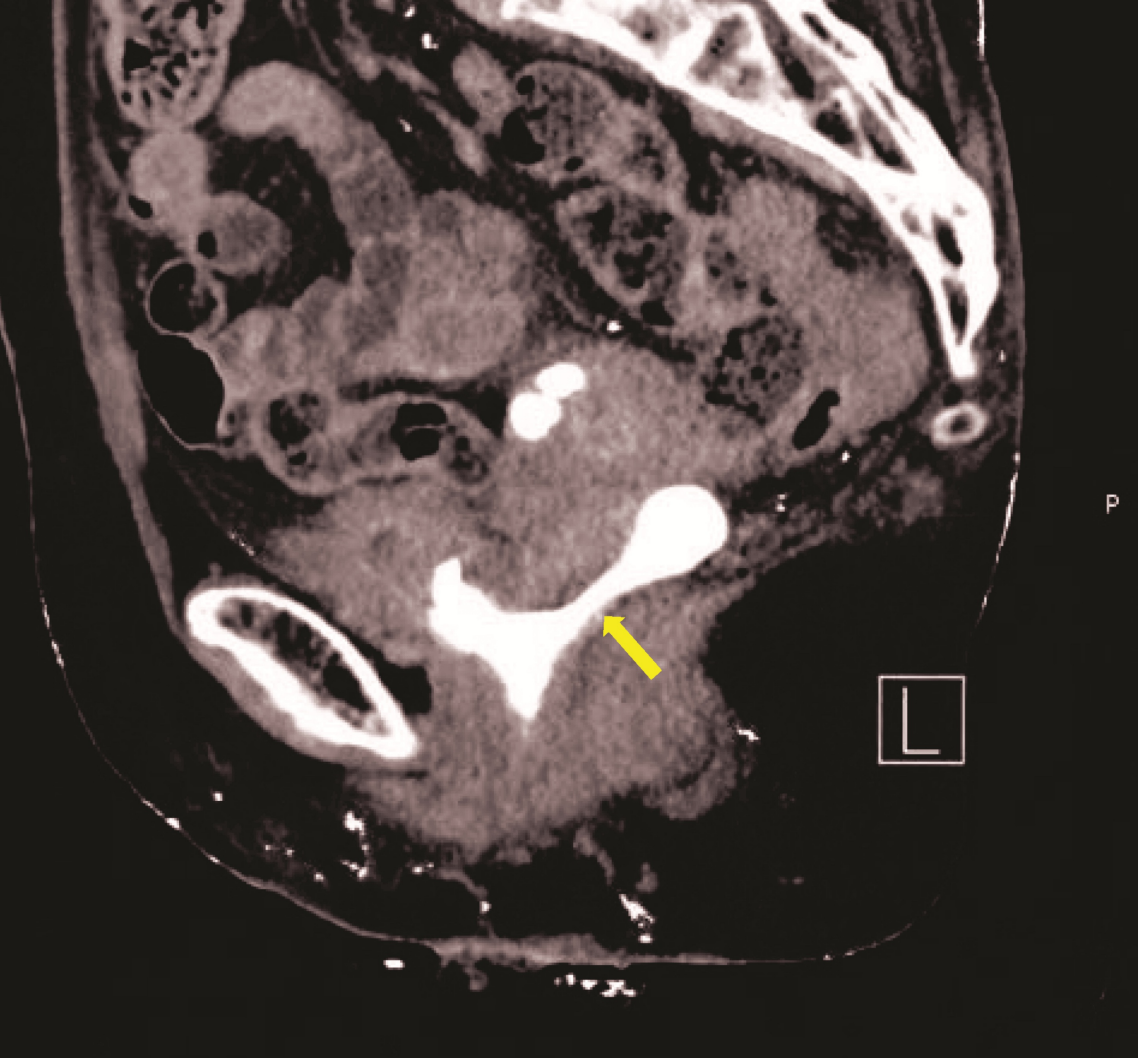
Excretory phase images of the contrast-enhanced CT. The contrast agent was stored from the bladder trigone into the vagina (arrow), suggesting a VVF. CT, computed tomography; VVF, vesicovaginal fistula
